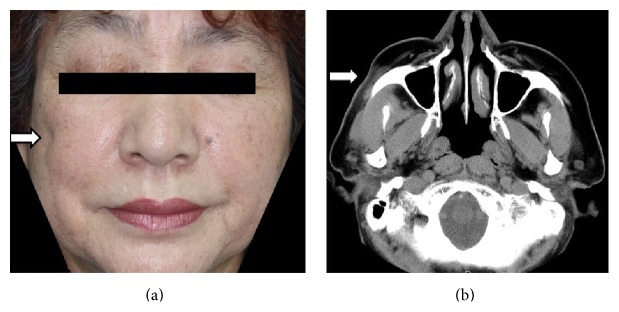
(a) Clinical manifestation at admission. (b) Axial computed tomography images, with soft-tissue settings, showing atrophy of subcutaneous fat on the right side of the face.
